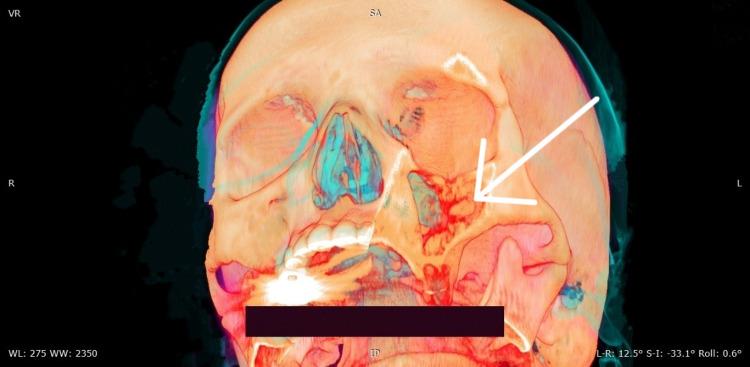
Reconstructed 3D CT image with supralateral angulation showing the undescended teeth in the left maxillary sinus 3D CT, three-dimensional computed tomography
